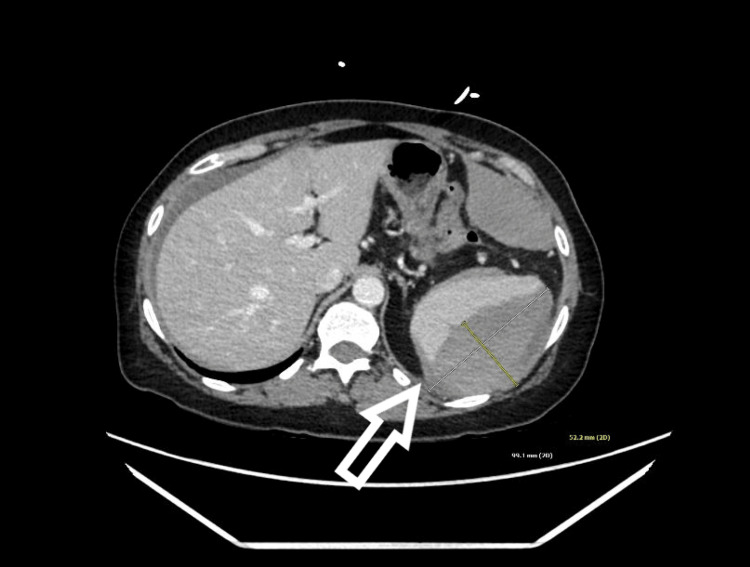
Transverse view of CT abdomen and pelvis with arrow pointing to splenic hematoma
